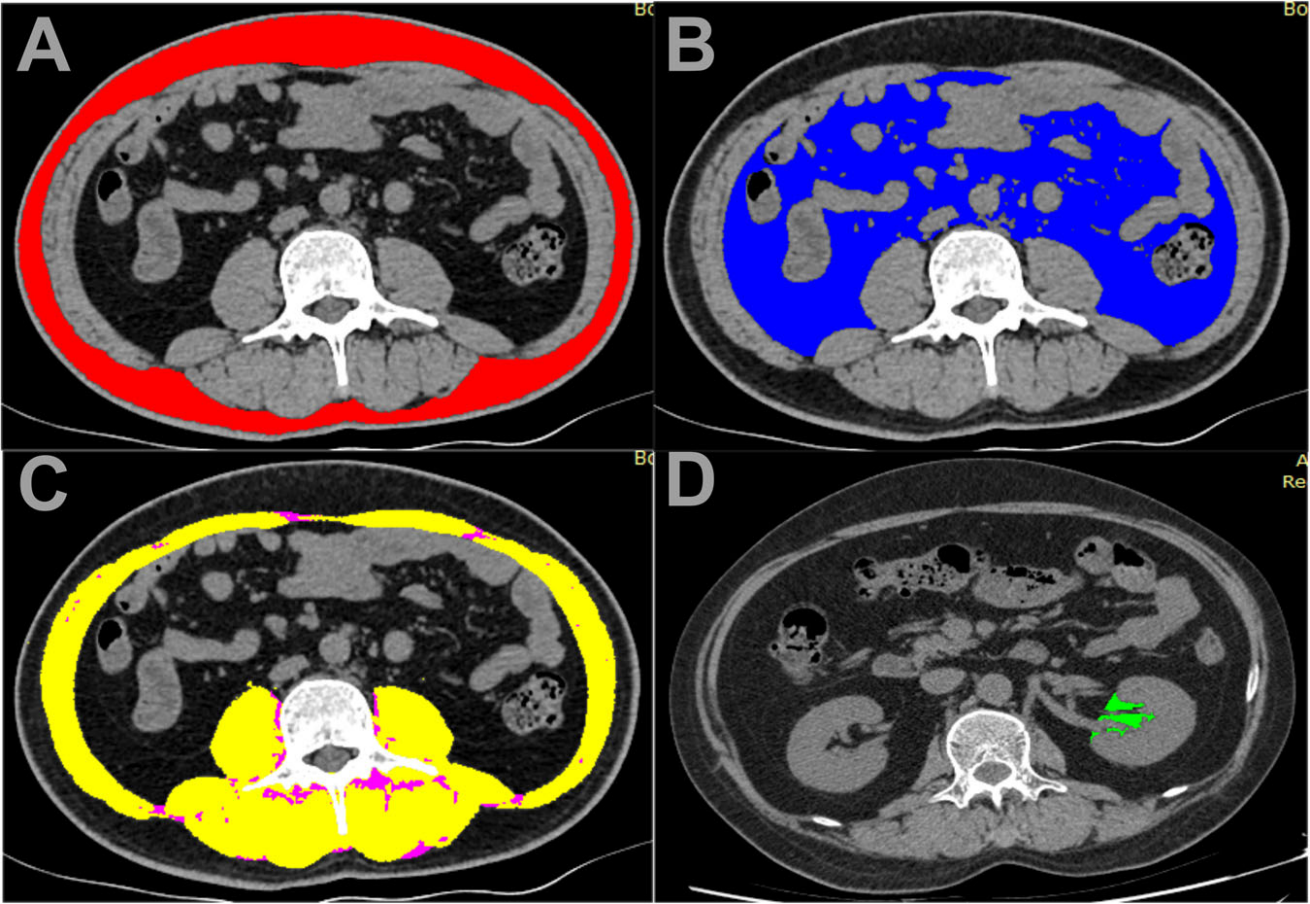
Representative cross-sectional CT images and muscle and fat of each part. A SAT (red); B VAT (blue); C SMA (yellow) and IIMAT (magenta); and D RSF (green)
